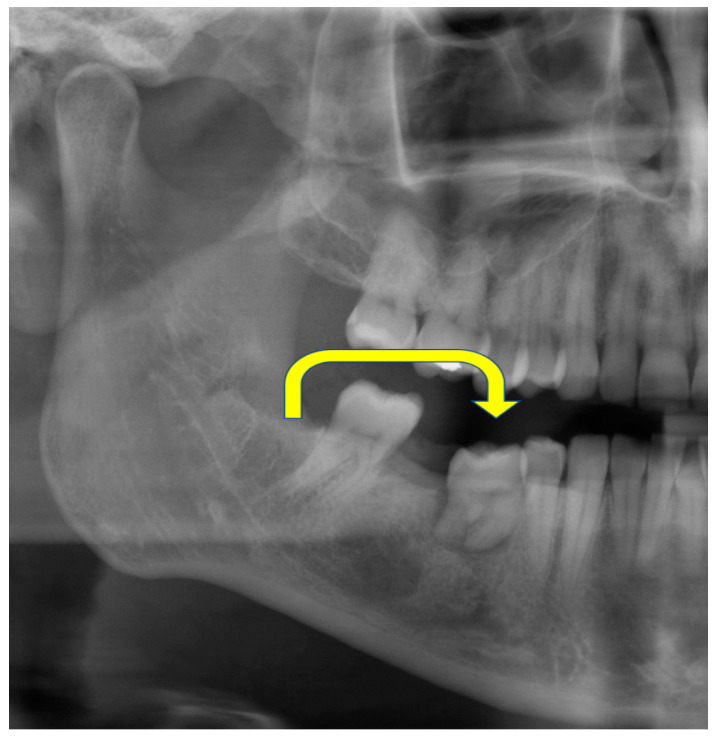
Postoperative panoramic radiograph. After removal of the deciduous tooth 85 the wisdom tooth and surgical preparation of the recipient socket tooth 48 had been transplanted (Yellow arrow). Note the shorter roots of the transplant after EORER.
